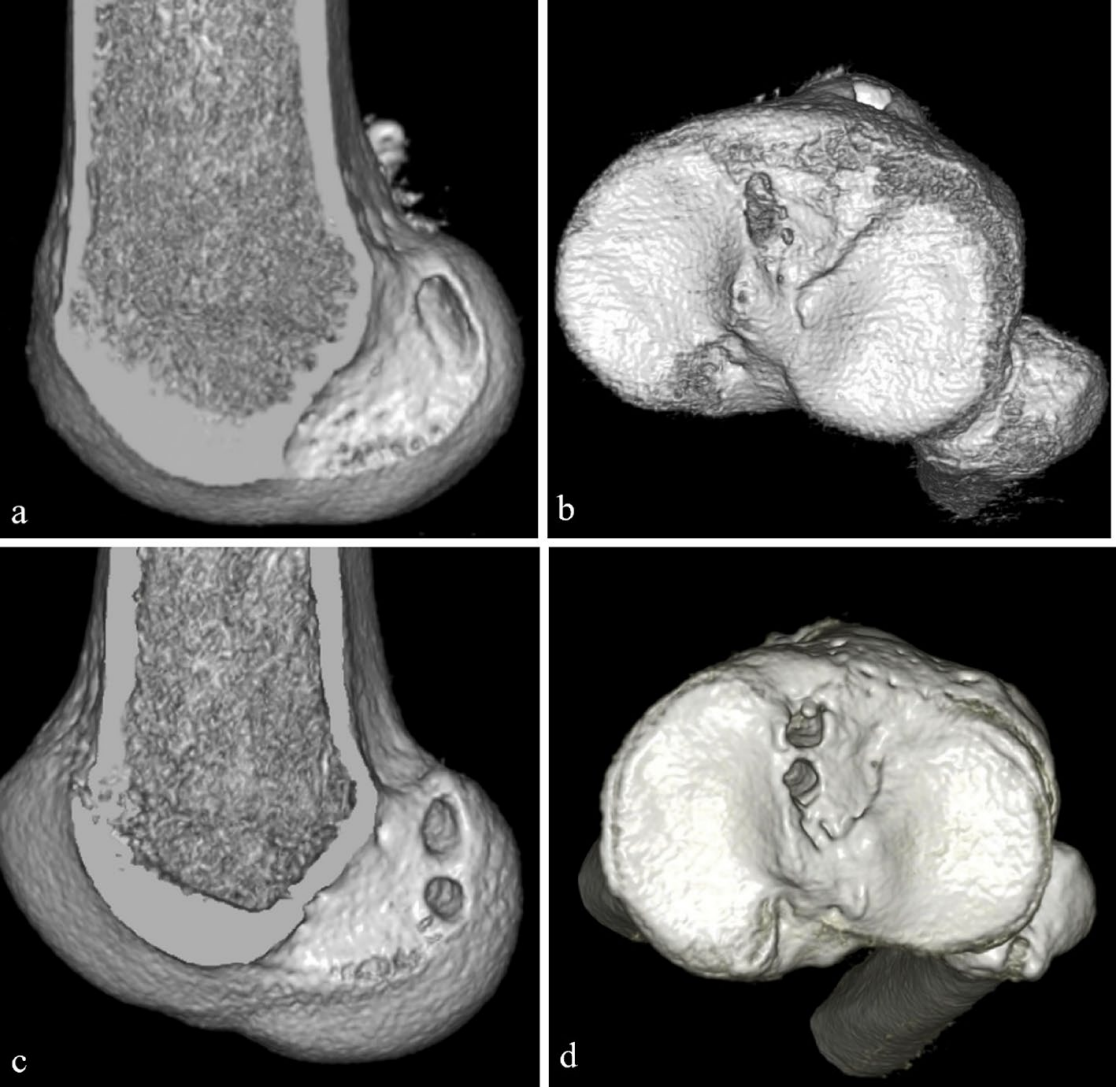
Representative tunnel positions on three-dimensional computed tomography images. a Femoral tunnel during ACL reconstruction with BPTB autograft. b Tibial tunnel with BPTB autograft. c Femoral tunnels with HT autograft. d Tibial tunnels with HT autograft. *ACL* anterior cruciate ligament, *BPTB* bone-patellar tendon-bone, *HT* hamstrings tendon
